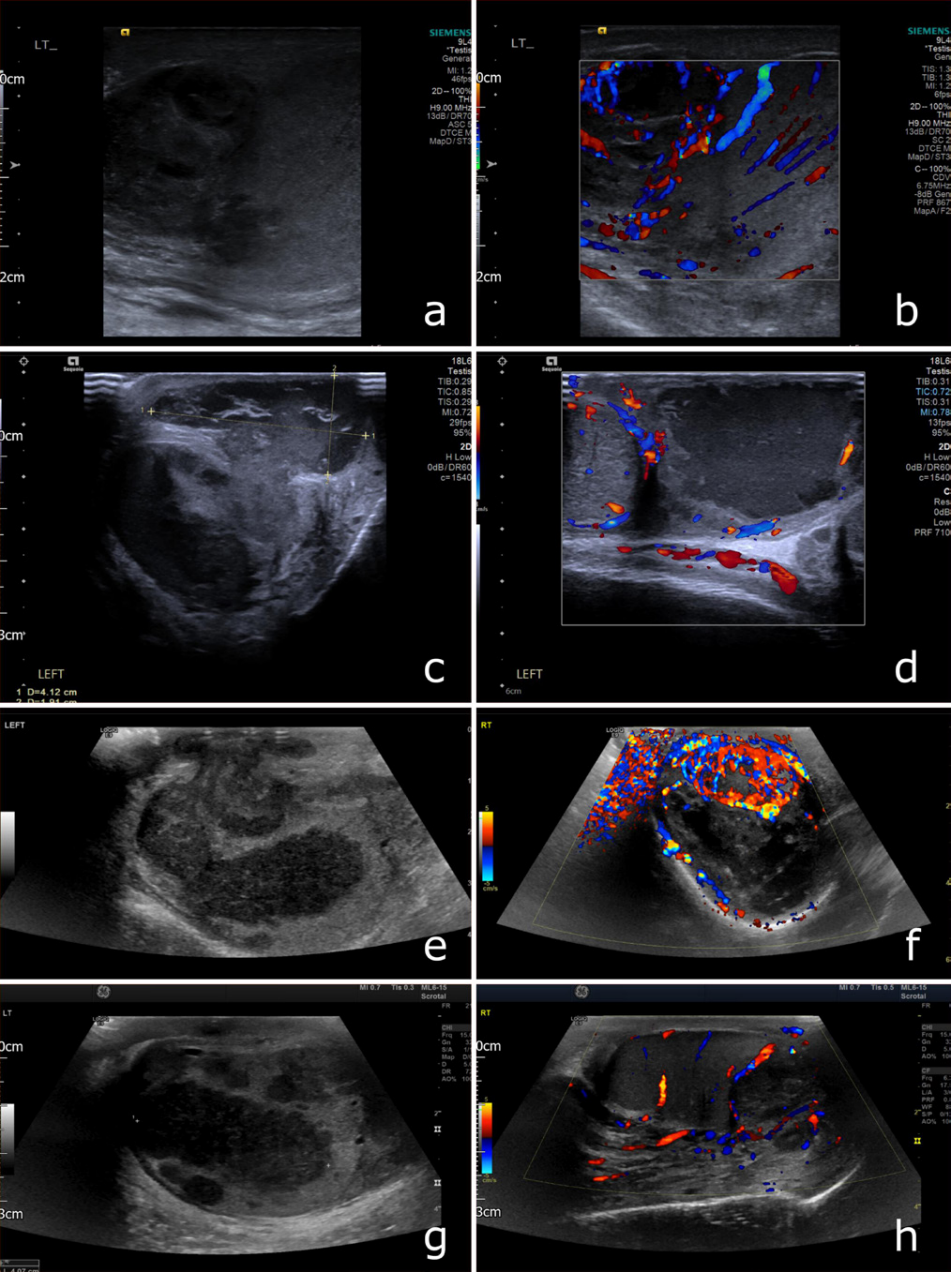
Ultrasound findings. (a) A large, heterogeneous, mass is seen centred on the head of the left epididymis at presentation. (b) The epididymal head and testis are hypervascular. (c, d) 3 weeks following the initial scan the lesion has progressed, extending further into the left testis; the right testis remains normal. (e) At 2 months, multifocal low attenuation lesions persist in the left testis. (f) There is also involvement of the right testis, with a floridly vascular lesion at the testicular hilum. (g, h) The testicular findings are similar at 3 months with less florid hypervascularity of the right testis. There is, however, new scrotal thickening (g) and discharge.
